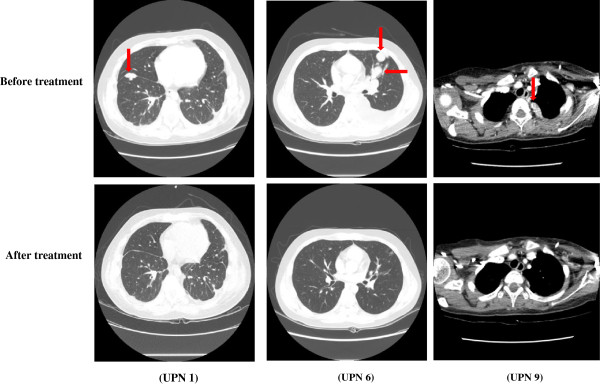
Computed tomography chest scans of three patients, showing response to docetaxel and irinotecan (DI) combination. No lung lesion was visible (complete response) after five cycles of DI in unique patient number (UPN) 1. UPNs 6 and 9 showed decreases in tumor (arrow) measurements of 40% after five cycles and 50% after three cycles, respectively, indicating partial responses by Response Evaluation Criteria in Solid Tumors (RECIST) version 1.1.
